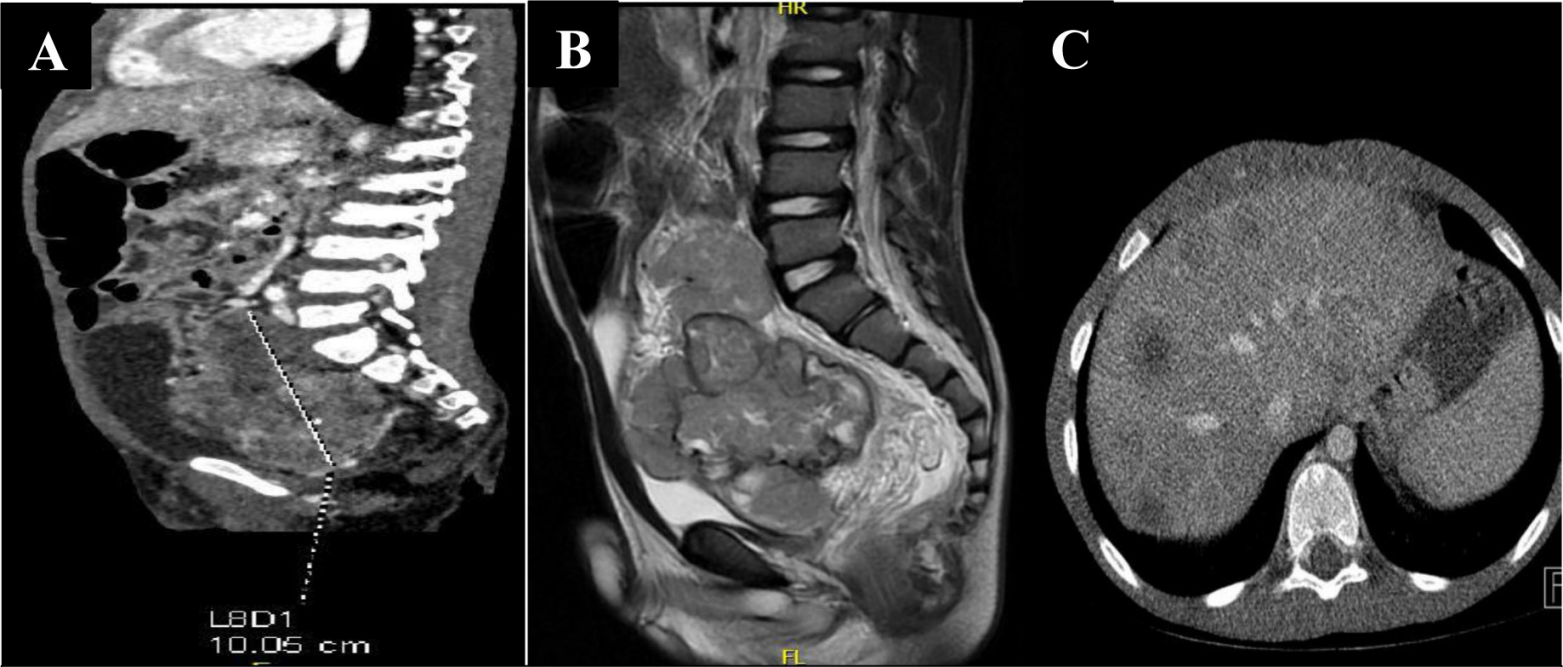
Sagittal CT image (A) shows a large mass with heterogeneous enhancement in the portal phase measuring 10 cm in maximum diameter, occupying almost the entire pelvis and extending up to L4–L5. Sagittal T2-weighted MRI (B) shows a large pelvic mass that correlates with the CT image. Axial CT image (C) confirms liver metastases.
